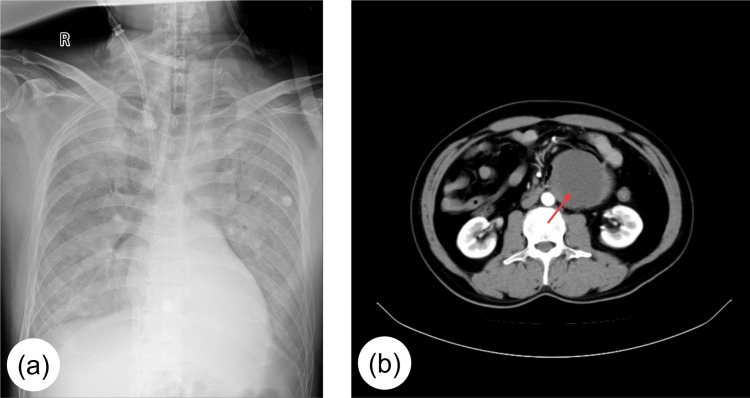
Imaging findings a: chest radiograph during ECMO support; b: abdominal CT scan showing the maximum axial diameter of the tumor (arrow). ECMO: extracorporeal membrane oxygenation
